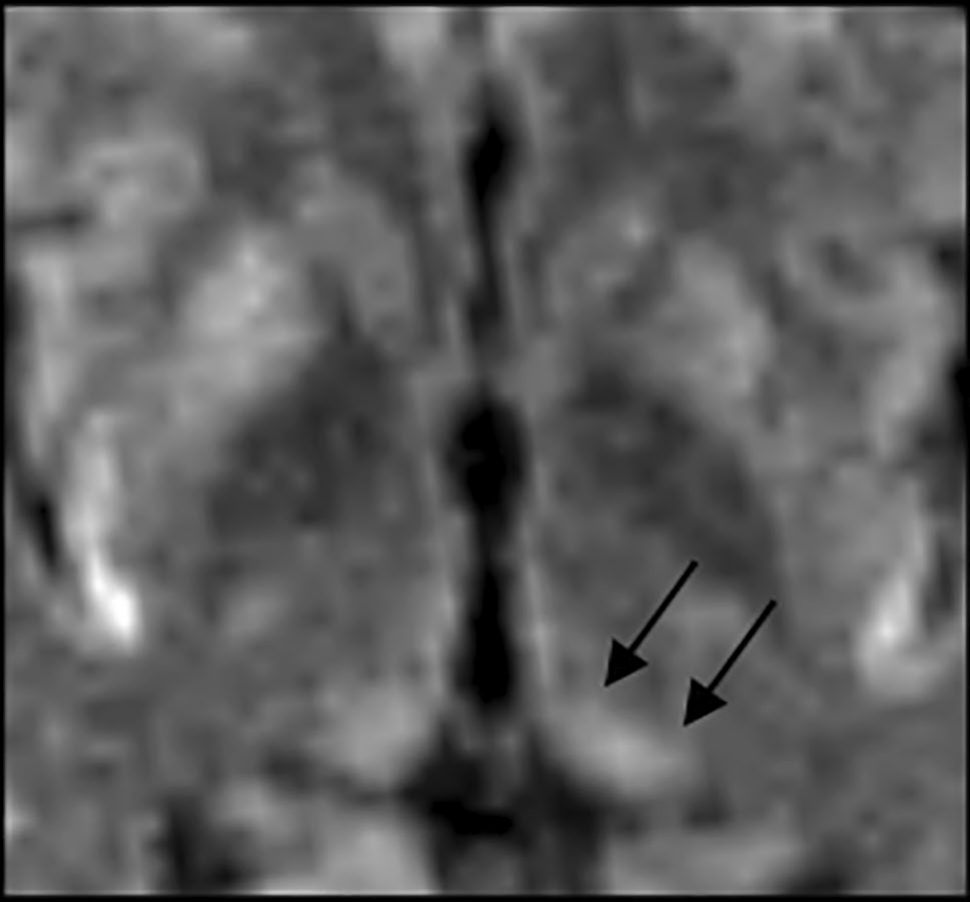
DWI-MRI axial image at the level of basal ganglia showing bilateral hyperintense pulvinar (arrows) in a 52-year-old patient with sCJD
